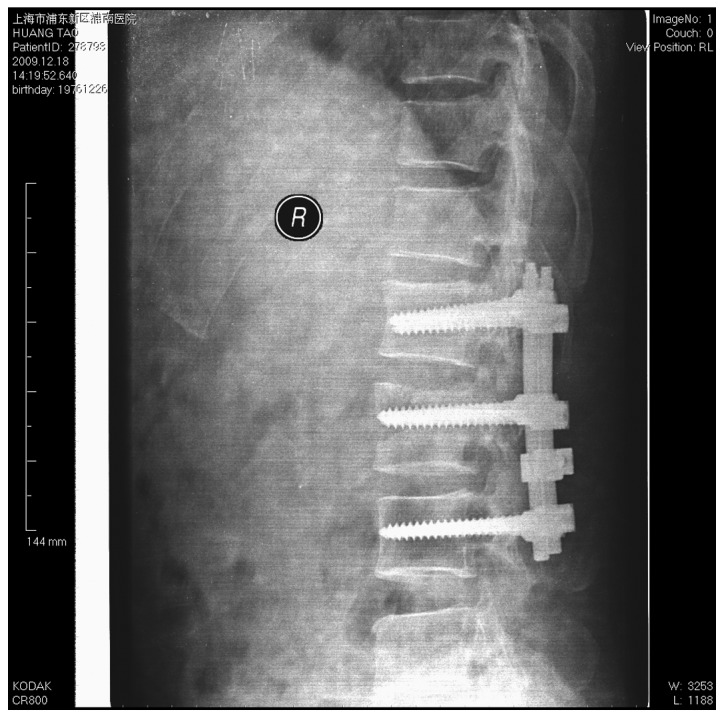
Lateral radiograph following triplane fixation of an L2 fracture for one year.
